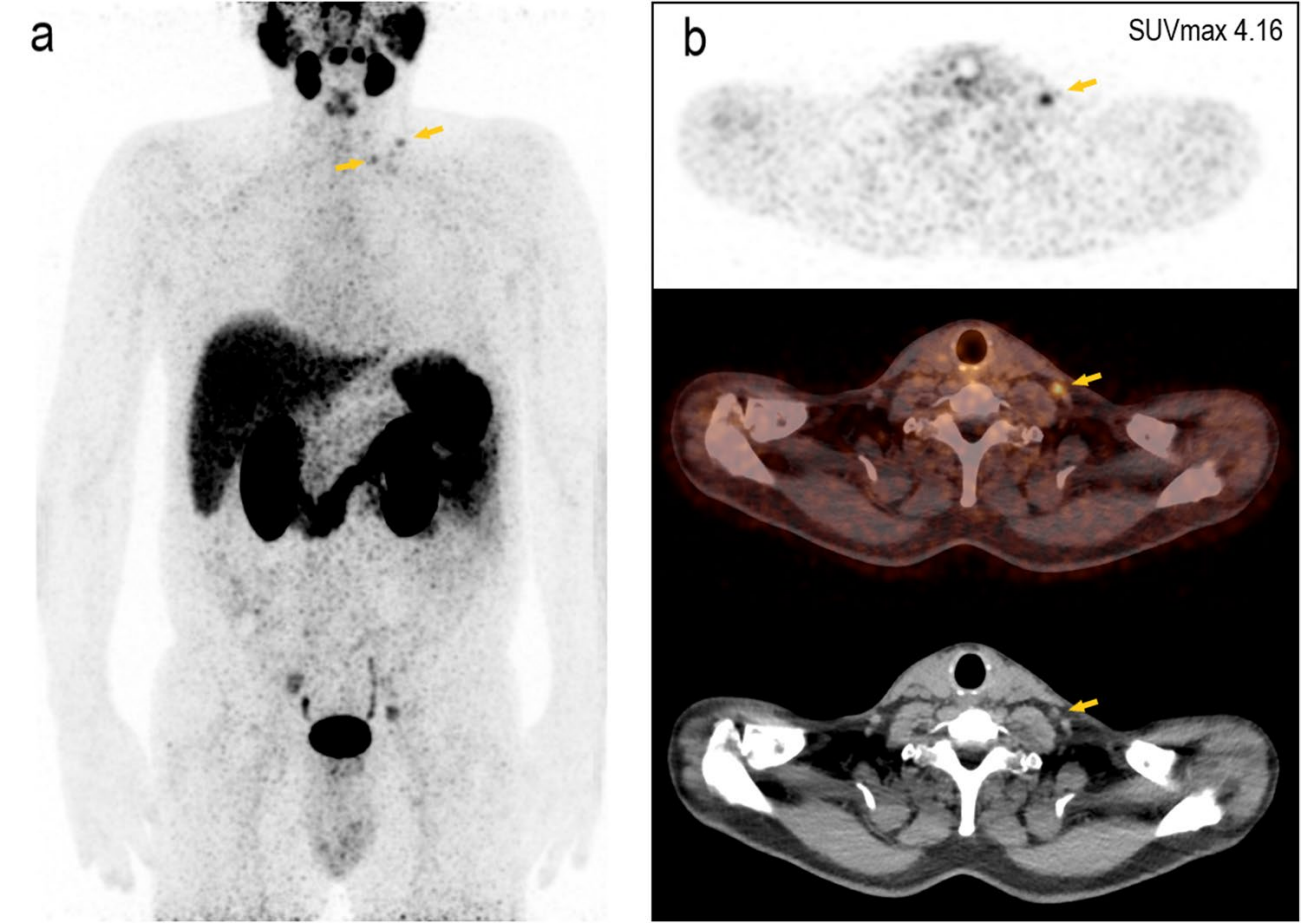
The potential of [^68^ Ga]Ga-PSMA-11 PET in the detection of distant LNM. A 59-year-old high-risk prostate cancer patient, Gleason score 7b, and tPSA of 11.99 ng/ml showed suspicious uptake in LN supraclavicular left. a Maximum intensity projection image; b PET image, fused PET/CT image, and CT image alone.
